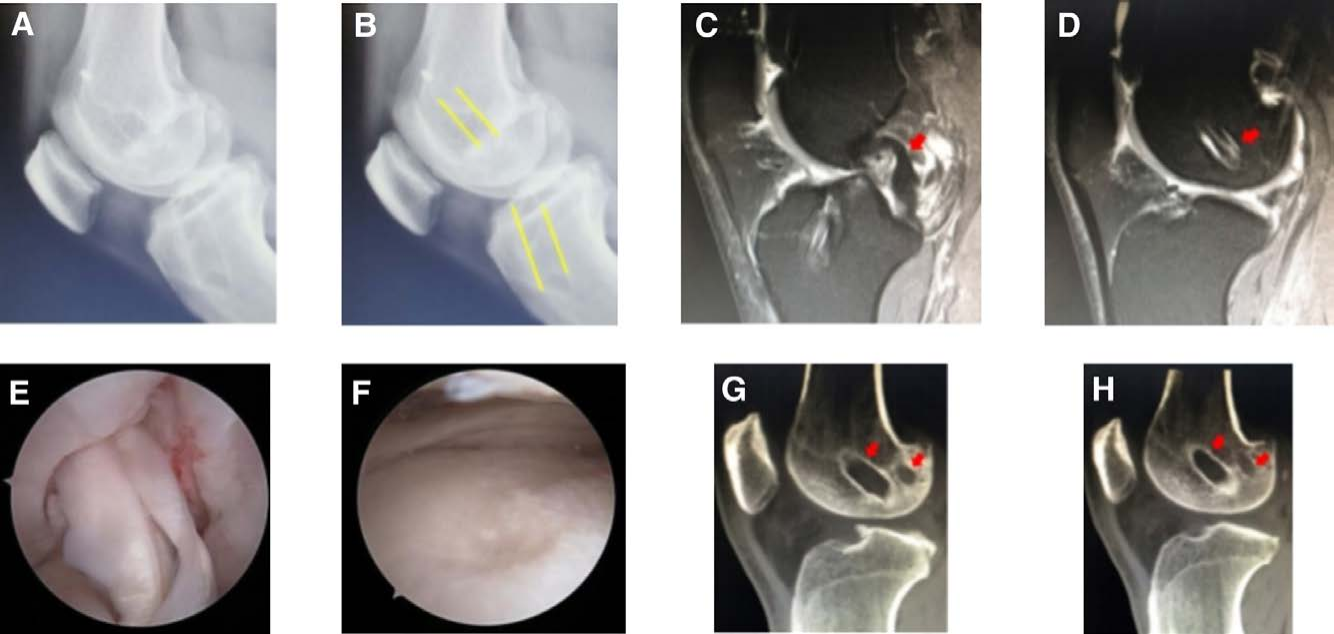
(A) DR: the tibial tunnel of the right knee was significantly enlarged, and the femoral tunnel was significantly anteriorly positioned. (B) The yellow line marks the location of the femoral and tibial tunnel, with the femoral tunnel situated anteriorly. (C and D) MRI findings: PCL relaxation exhibited a question mark configuration, ACL graft relaxation exhibited signal disorder, and the femoral tunnel was positioned significantly anterior. (E and F) Intraoperative findings: hyperosteogeny in the intercondylar fossa, ACL graft relaxation, and total knee degeneration. (G and H) CT image: reconstruction of the anatomic bone canal was performed post-operation. ACL = anterior cruciate ligament, PCL = posterior cruciate ligament.
